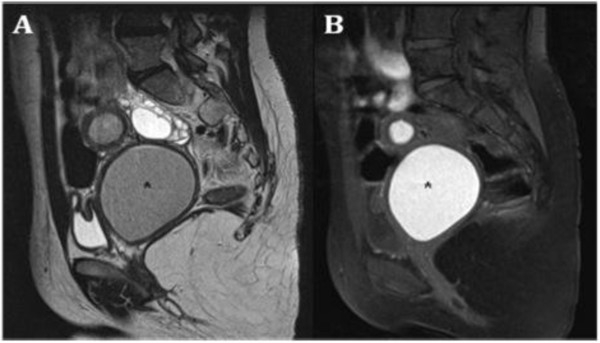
A) Sagittal T2-weighted MR image demonstrates an enormously dilated hemivagina (asterisk) due to the presence of the transverse septum causing the obstruction and low T2-signal-intensity contents corresponding to a high T1-signal intensity; B) consistent with blood product due to the presence of haemoglobin.
